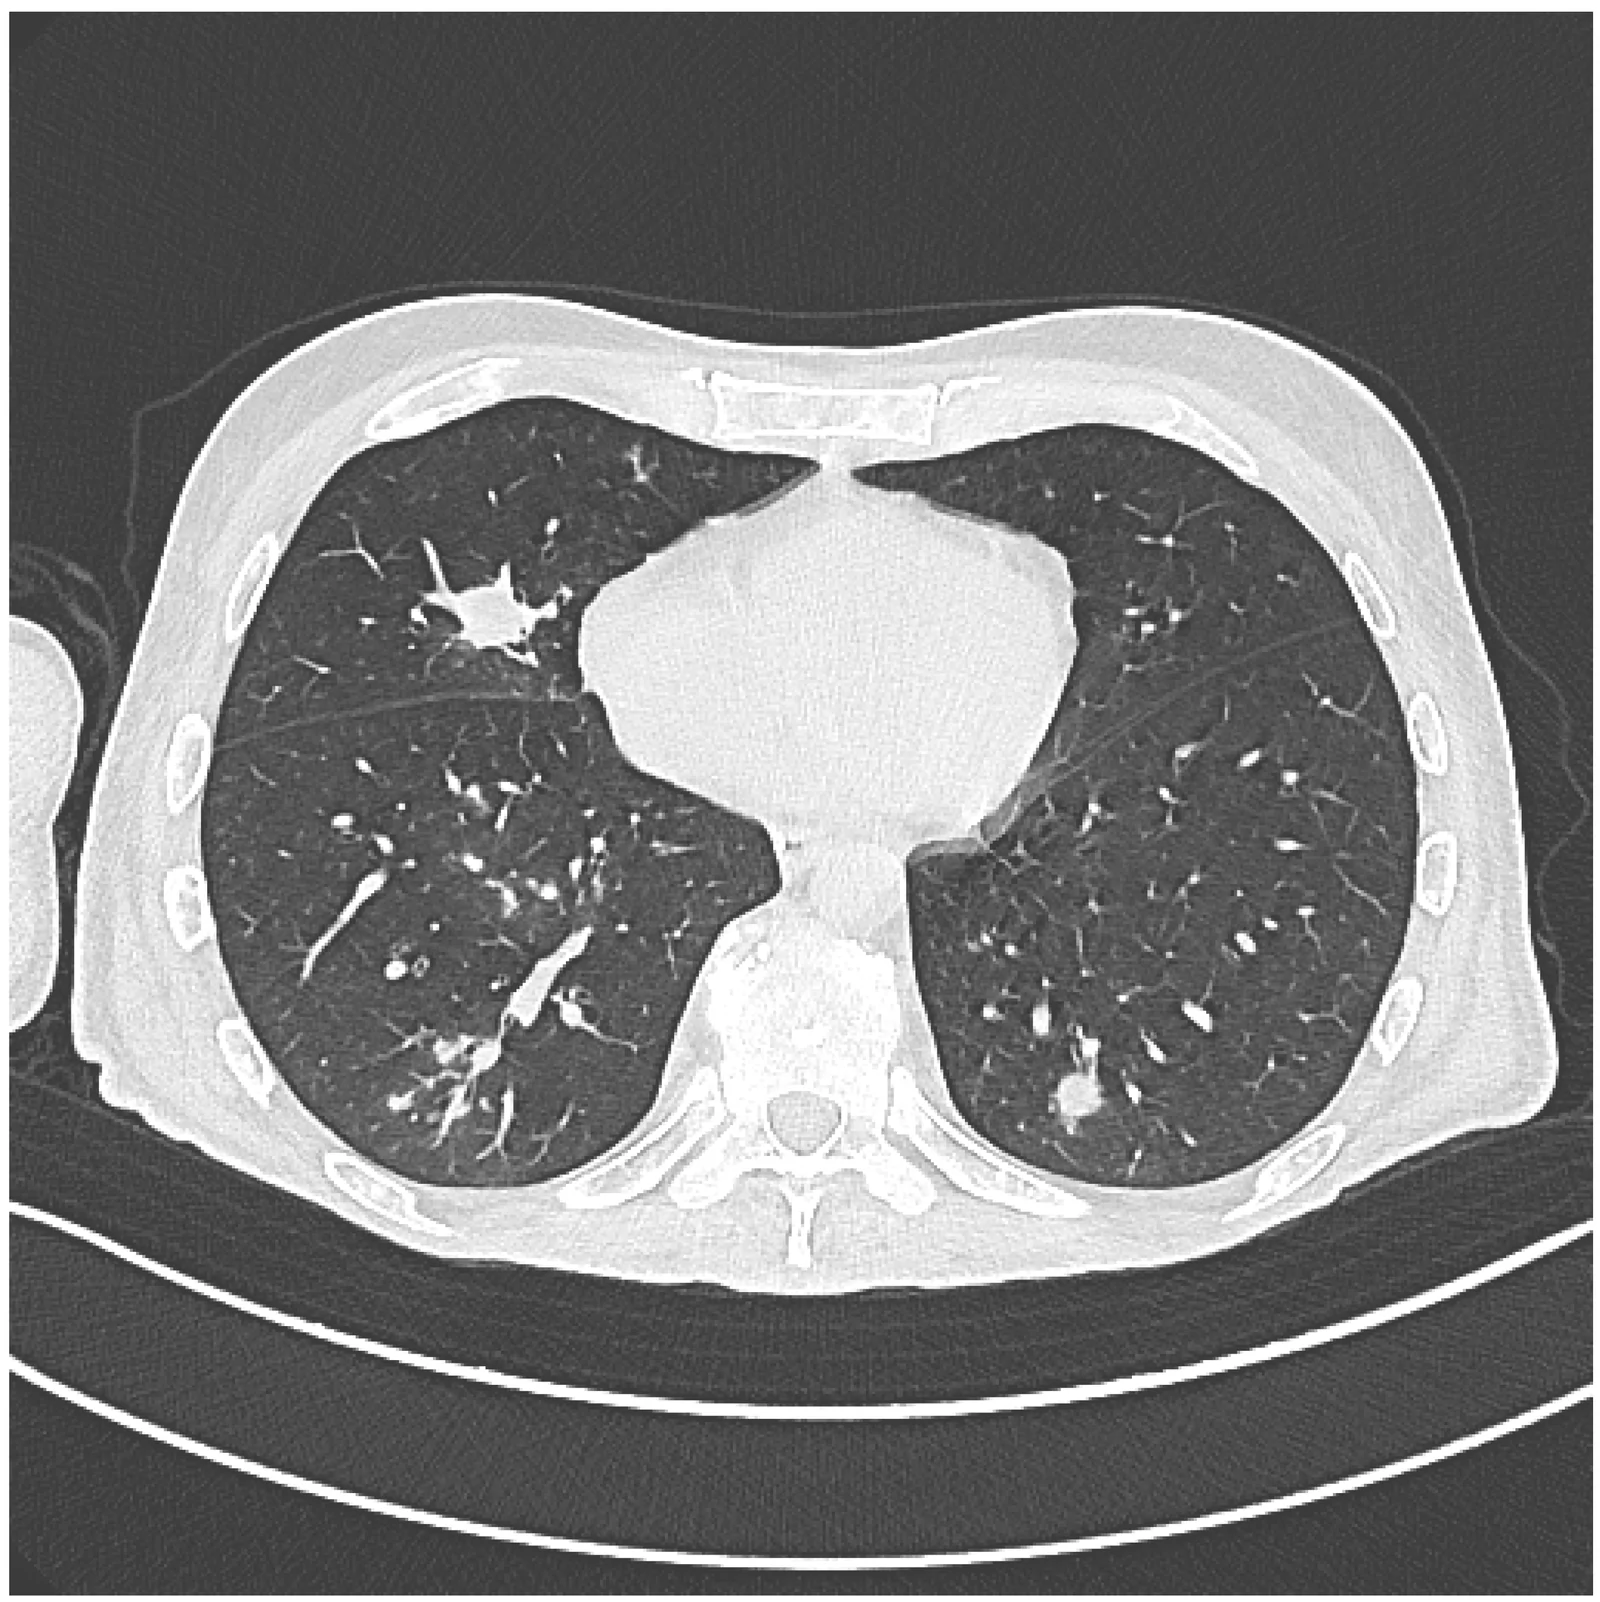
HRCT, axial plane. HRCT scans reveal multiple bilateral nodular opacities, prevalent in peripheral regions. Pulmonary tularemia in this case mimic neoplastic lesions, but in the right lower lobe the findings are more indicative of an infectious disease.
